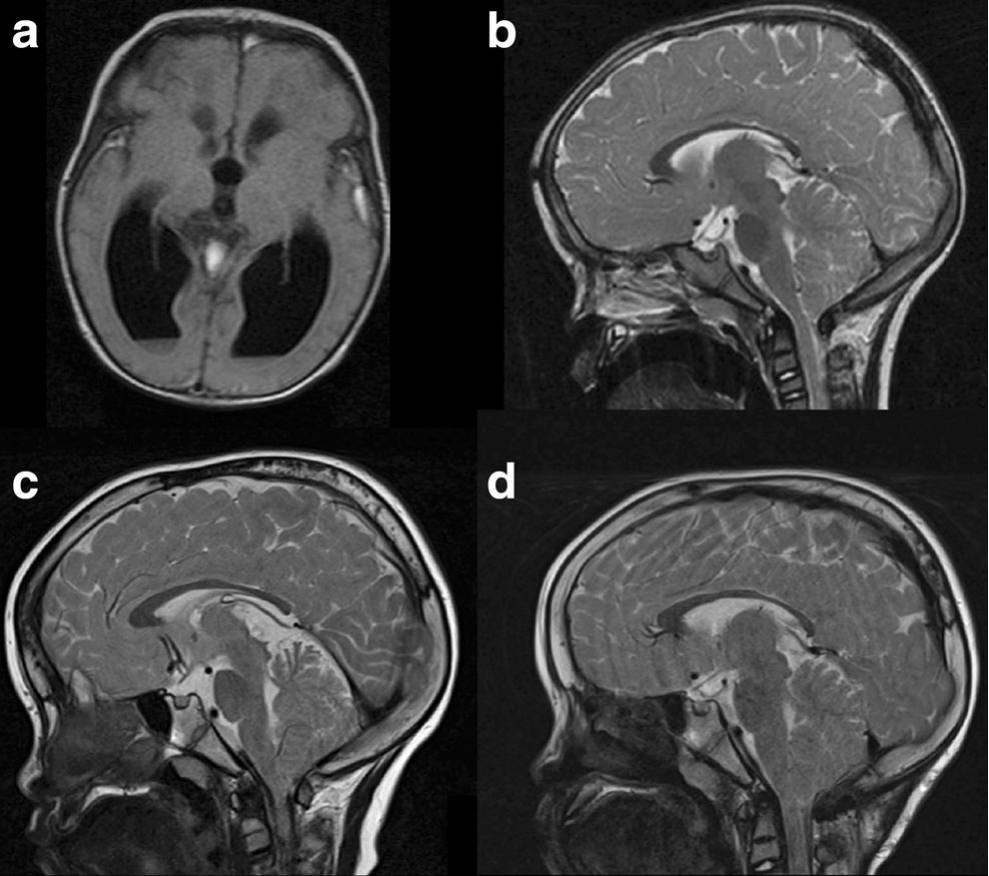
Case 3. MRI scan of a bleeding from a cyst in the right thalamus (a), treated with fenestration of the cyst, external ventricular drainage, and eventually a ventriculoperitoneal shunt. During follow-up, an asymptomatic Chiari I malformation was seen (b), that became symptomatic after a clear thickening of the cranial vault in the following years (c). The postoperative MRI scan shows an adequate decompression after a C1 laminectomy and posterior fossa decompression, during which we placed back the thinned occipital plane after remodeling it to a normal size (d)
